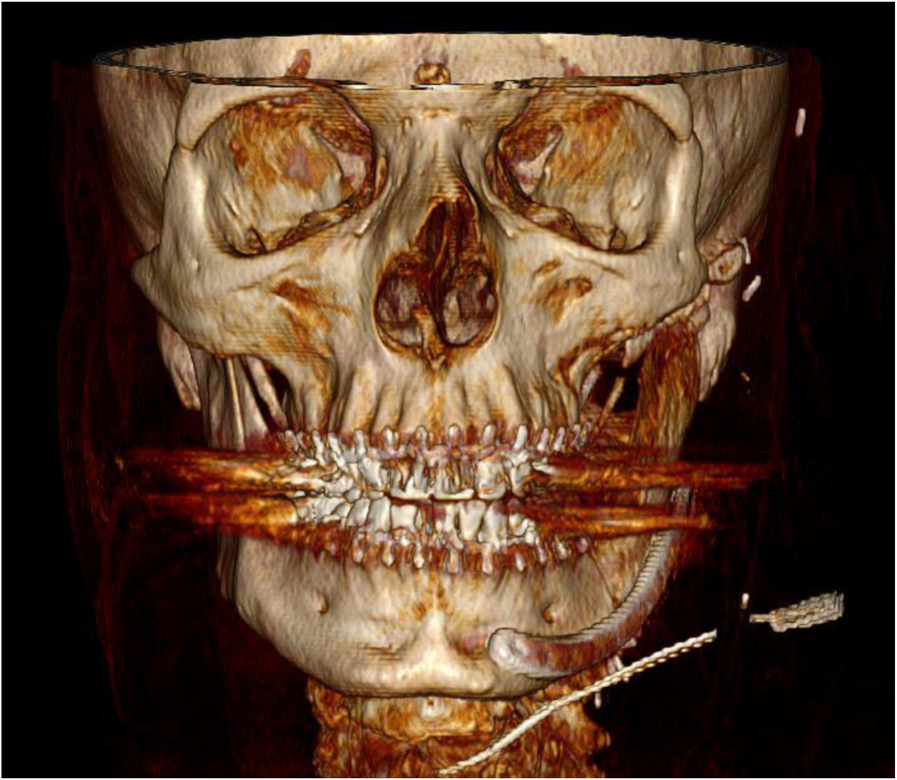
Postoperative CT scan of patient 3
